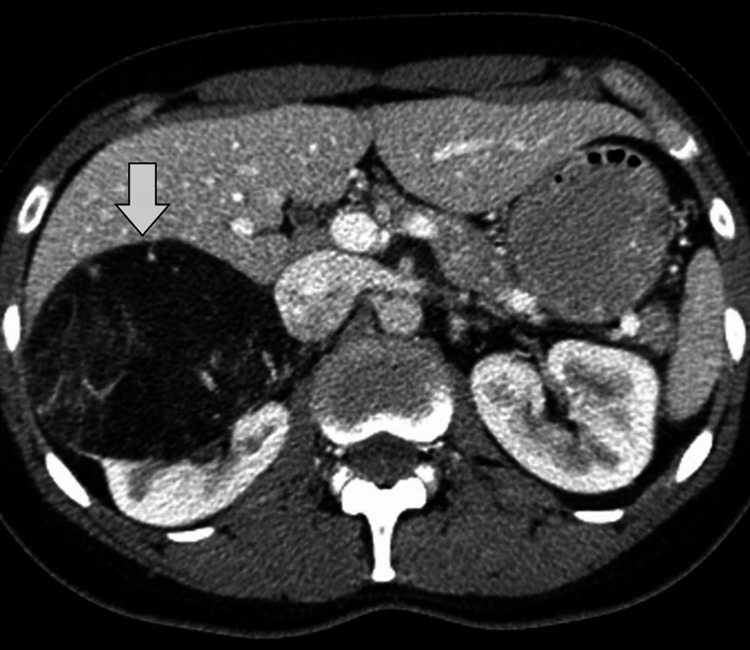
Renal angiomyolipoma on computed tomography Axial contrast-enhanced computed tomography (CT) image of the abdomen demonstrates a well-defined fat-containing lesion (arrow) arising from the right kidney, characterized by areas of negative attenuation consistent with renal angiomyolipoma.
